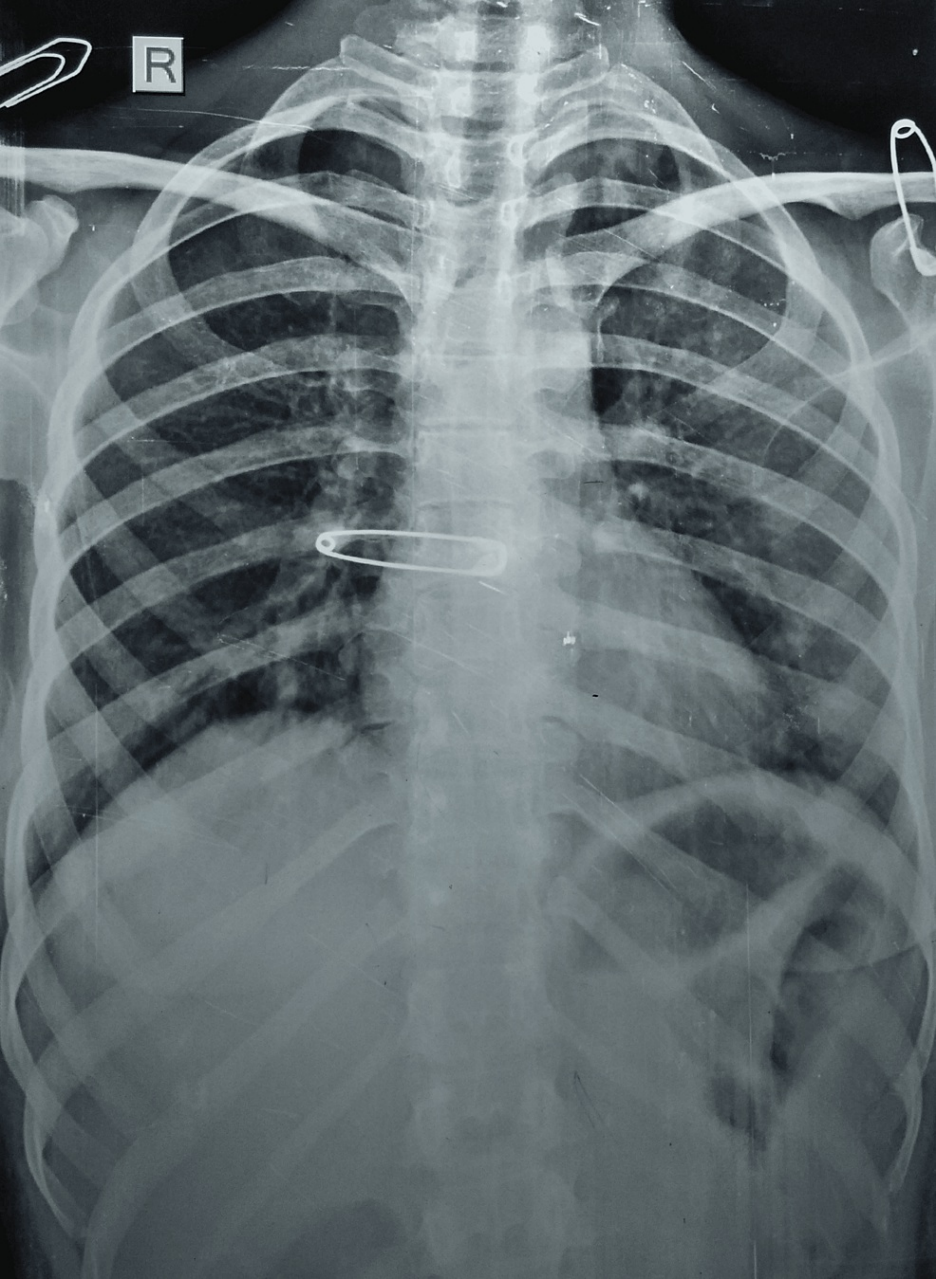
A chest radiograph (P-A view) not suggestive of tuberculosis P-A: postero-anterior
